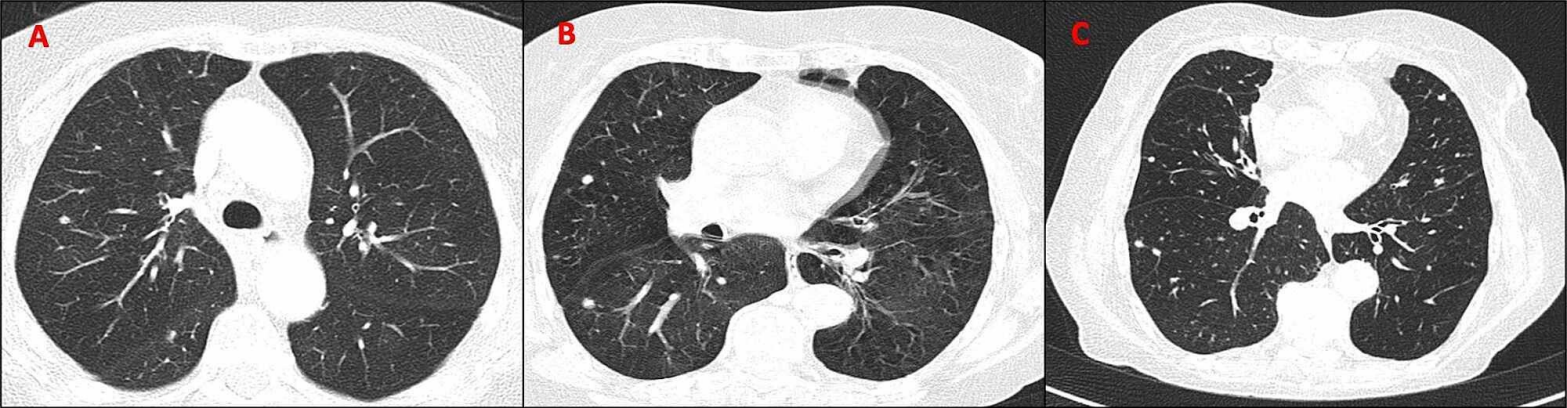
Chest CTs from 2008 (Panel A), 2011 (Panel B), and 2018 (Panel C) showing the progression in size and distribution of a parenchymal micronodular pattern. CT, computed tomography
